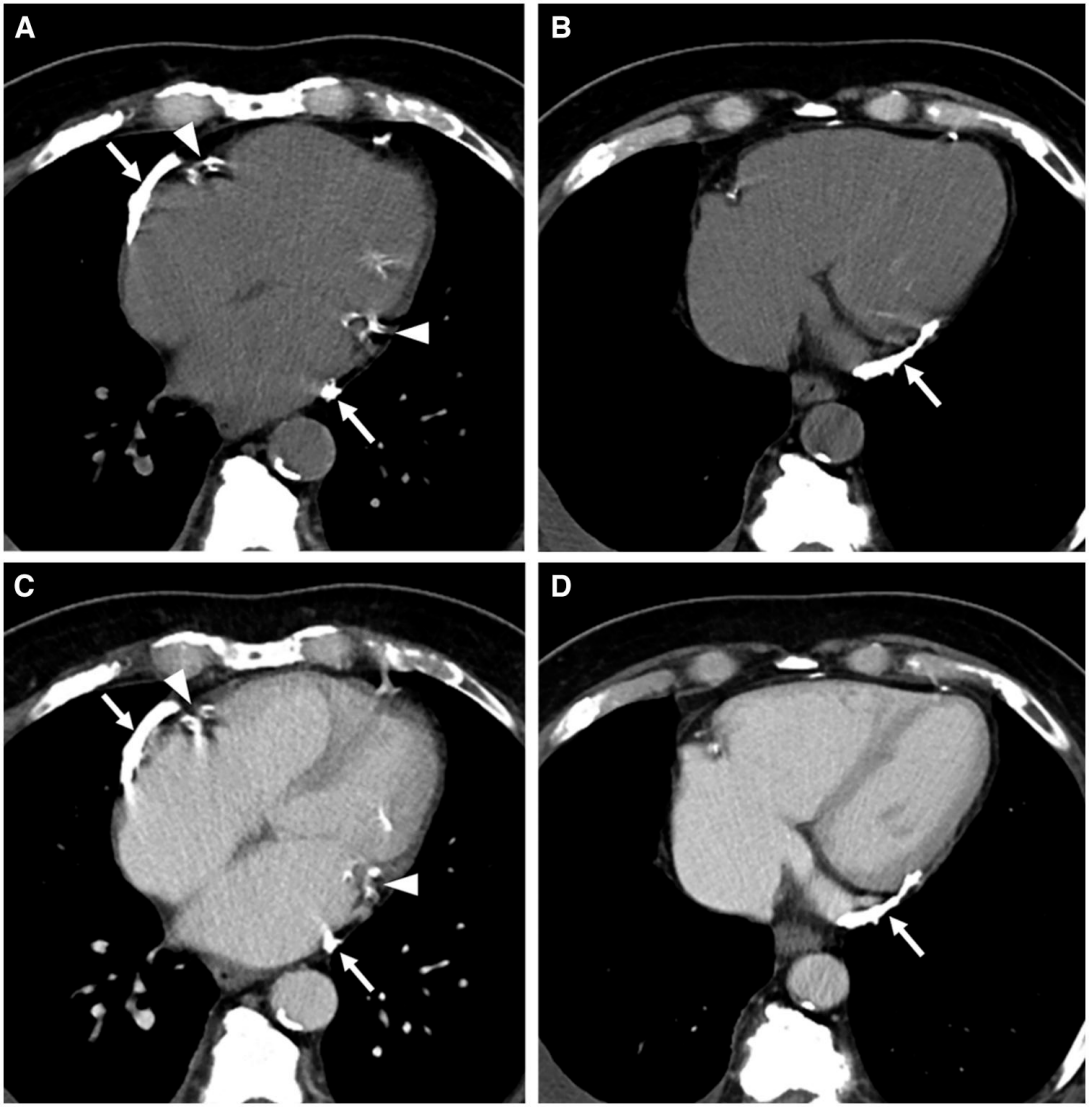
Tuberculous calcific pericarditis in a 79-year-old man. Axial (A and B) unenhanced and (C and D) contrast-enhanced CT images taken at 2 corresponding levels show pericardial calcifications (white arrows) along bilateral atrioventricular grooves with dilatation of right and left atria (bi-atrial enlargement), suggestive of constrictive pericarditis. Note the calcified coronary arteries from atherosclerosis (white arrowheads).
